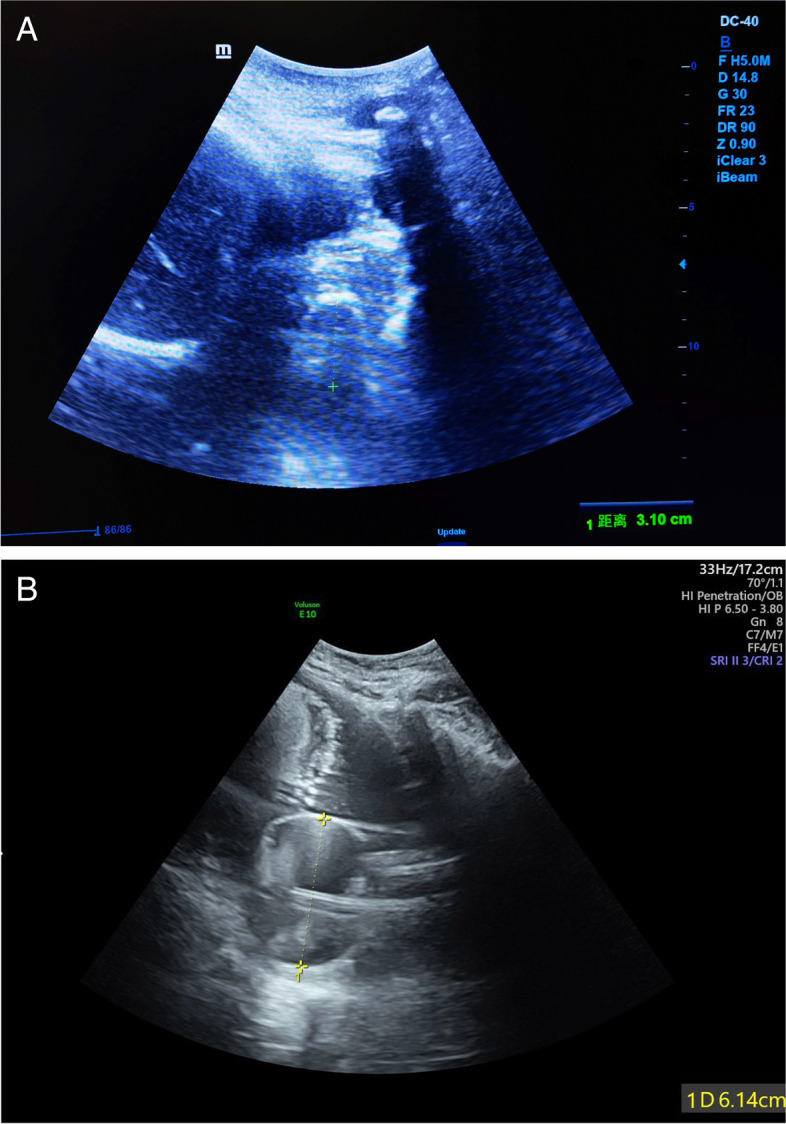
Ultrasonic images of the uterine balloon with different volumes. A: Control group; B: Study group
